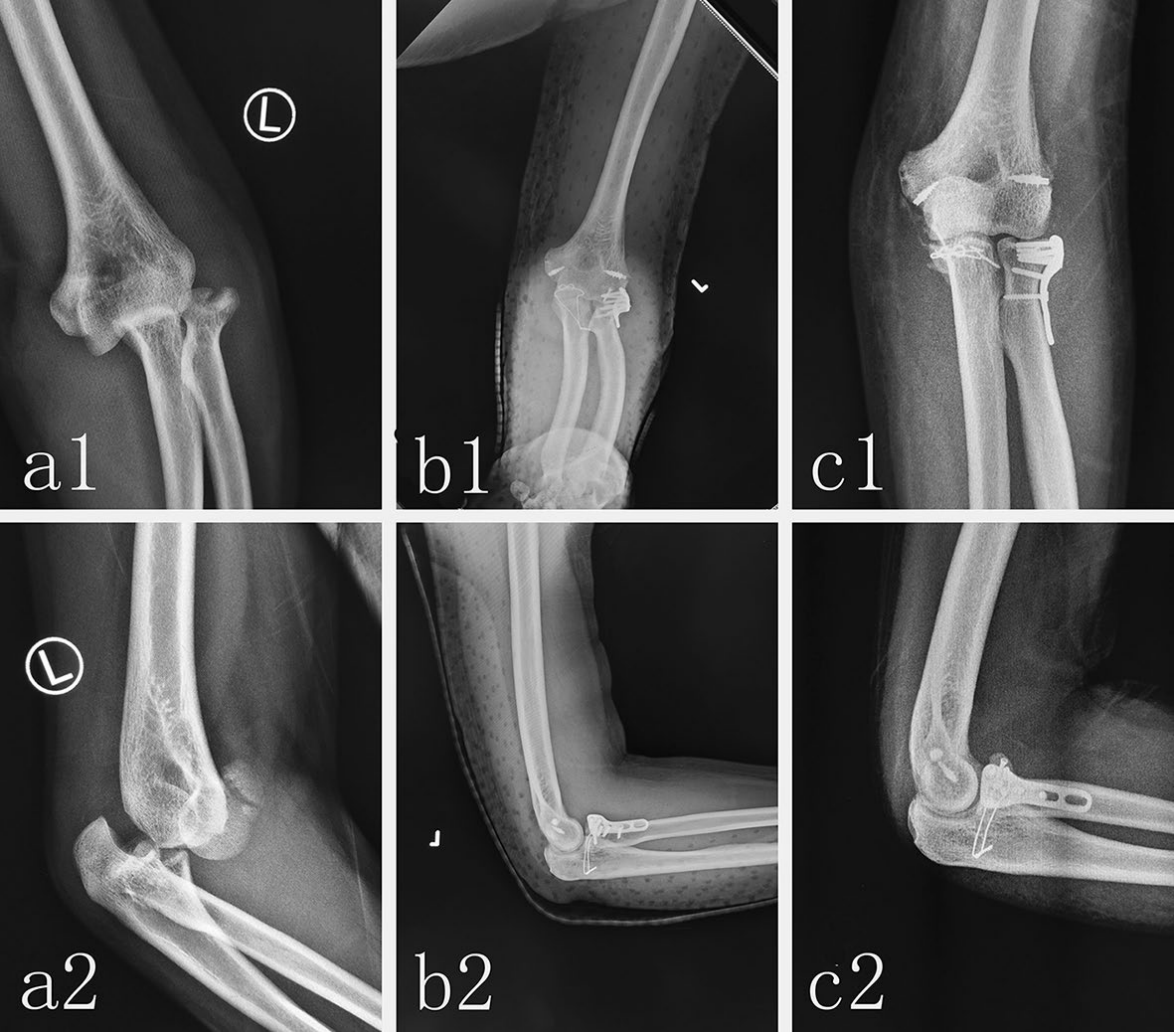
Radiological image of typical patient with TTE using Lasso-plate technique. The preoperative anteroposterior X-ray (a1) and lateral X-ray (a2) showed the posterior dislocation of left elbow joint, the fracture of radial head and coronoid process. An anteroposterior X-ray (b1) and lateral X-ray (b2) at postoperative follow-up showed that the fracture of coronoid process was reduced and fixed by Lasso-plate technique, radial head fracture was fixed by miniplate, and the LCL of elbow were repaired by suture anchor. An anteroposterior X-ray (c1) and lateral X-ray (c2) at 6-months postoperative follow-up showed that fracture had healed well and the dislocation and instability of elbow were not observed.
